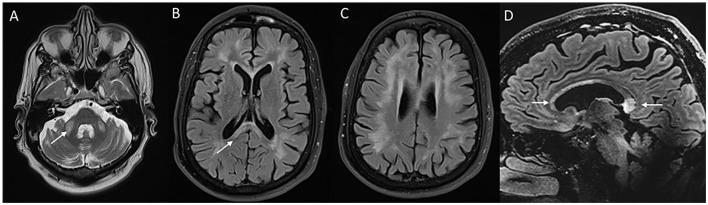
Neuroimaging in fragile X-associated tremor/ataxia syndrome. MRI T2W axial (A) reveals symmetric ovoid lesions in the middle cerebellar peduncle, along with prominent foliae suggestive of diffuse cerebellar atrophy. T2 FLAIR axial sections (B, C) show signal changes in the subcortical and white matter and splenium [arrow in (B)]. FLAIR sagittal section (D) shows anterior callosal and splenial hyperintensity.
